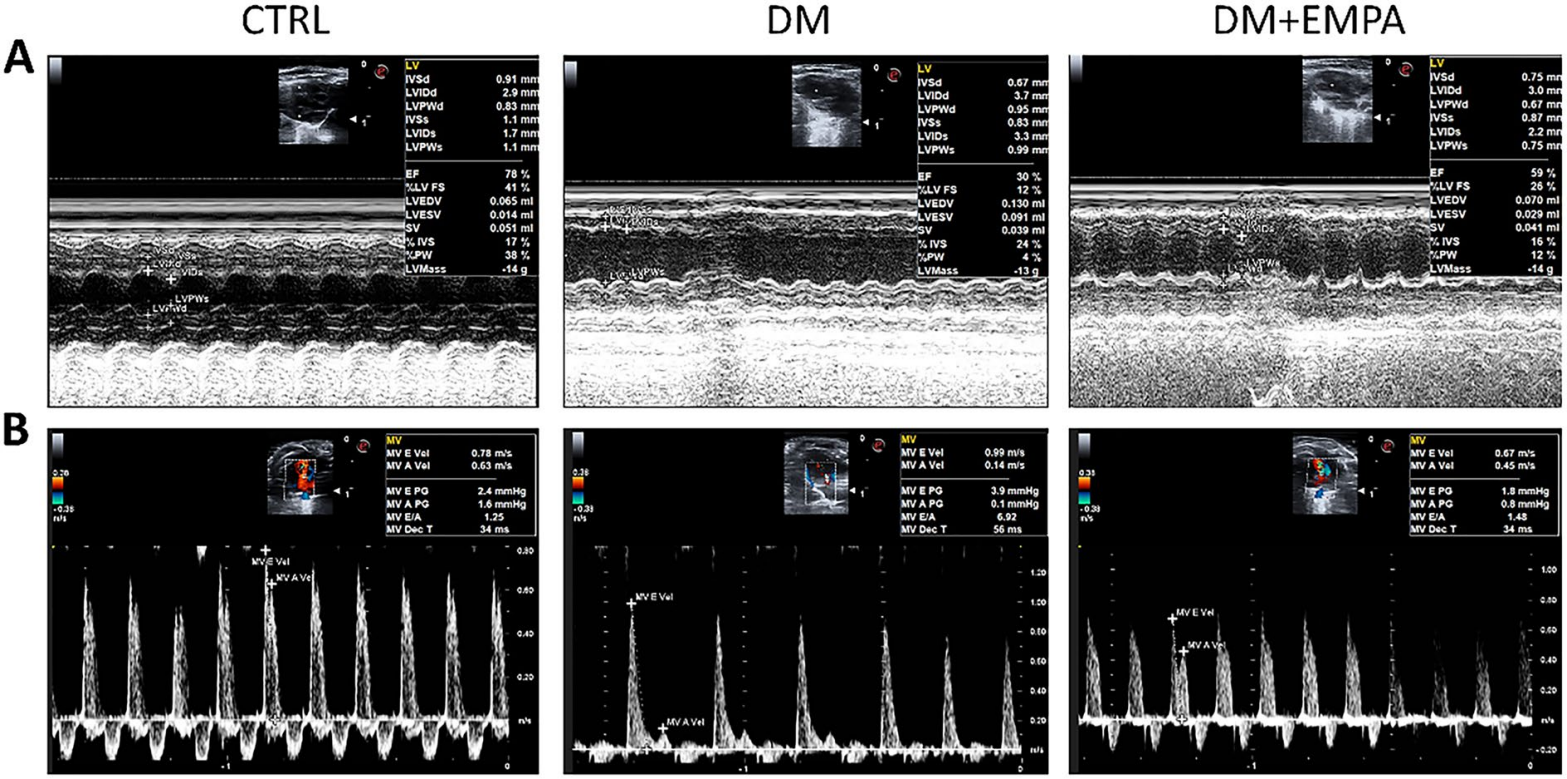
The structure and function of the hearts were examined by ultrasound. A showed the structural parameters of the hearts. B showed the diastolic function of the hearts
